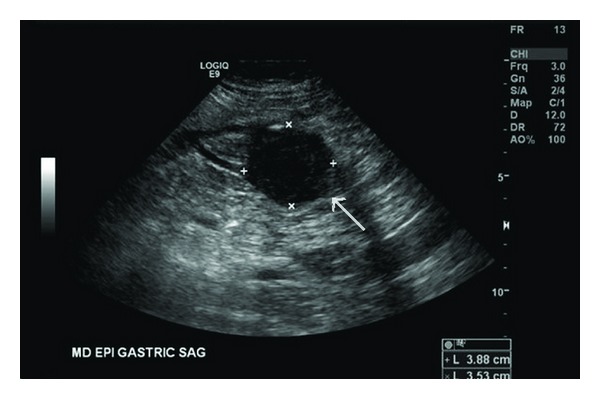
Transabdominal sonogram reveals a round and well-defined mass in the stomach.
